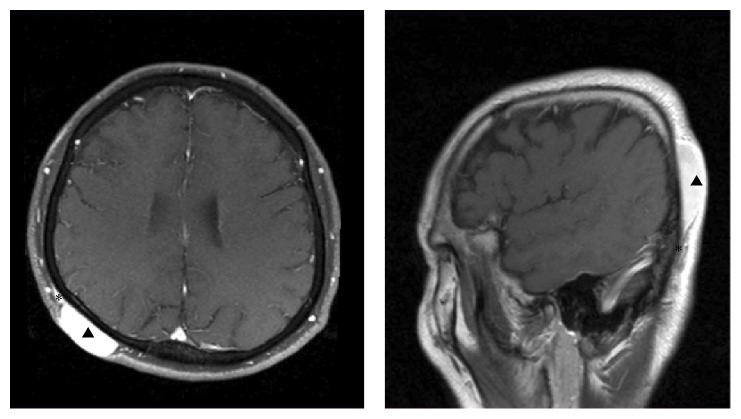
MRI image showing the subcutaneous extension of DFSP. These images help to determine the outline of DFSP. Arrow heads present primary lesion of DFSP in scalp. Asterisks present subcutaneous extension beyond the macroscopic tumor margin. MRI: magnetic resonance imaging, DFSP: dermatofibrosarcoma protuberans.
